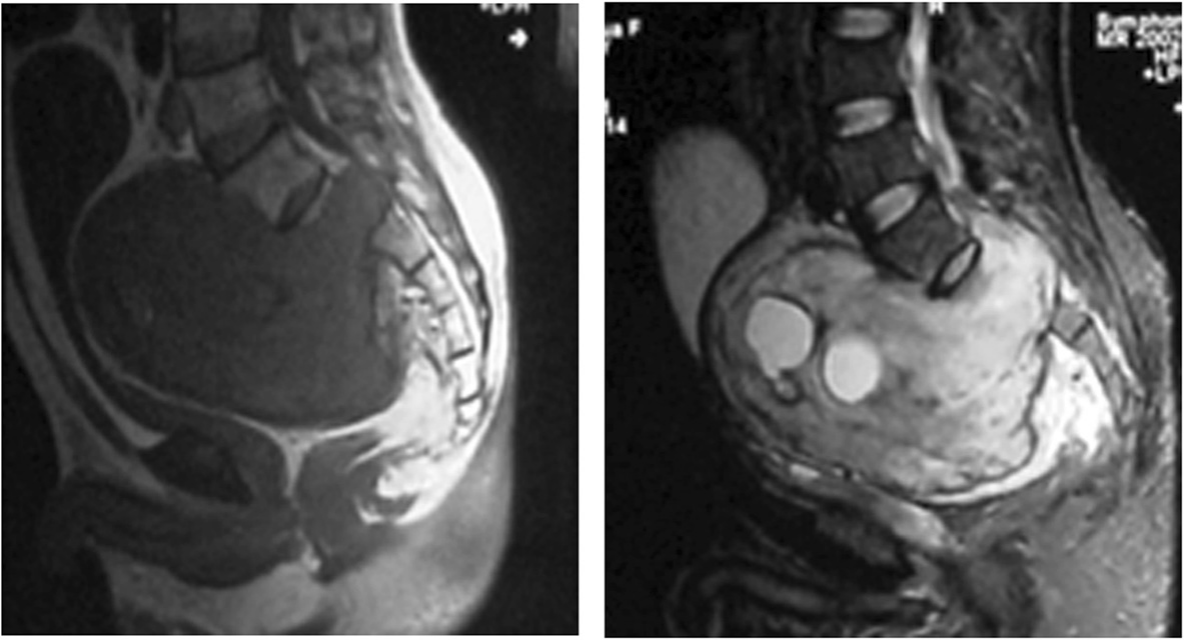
The magnetic resonance imaging (MRI) of patient C after gelatin sponge particle (GSPs) implantation taken in July 2014
